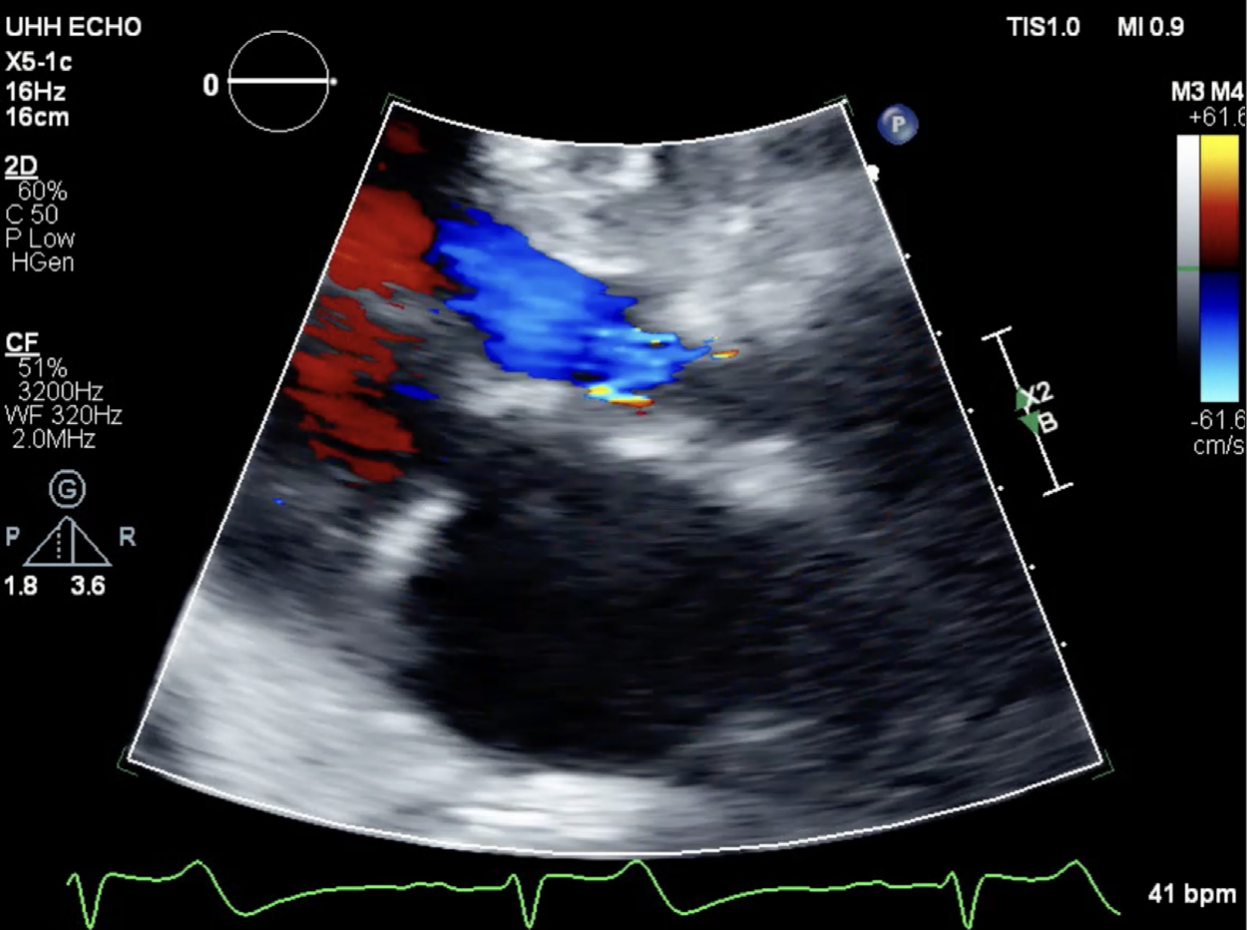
Cardiac transthoracic echocardiography demonstrating severe aortic regurgitation.
